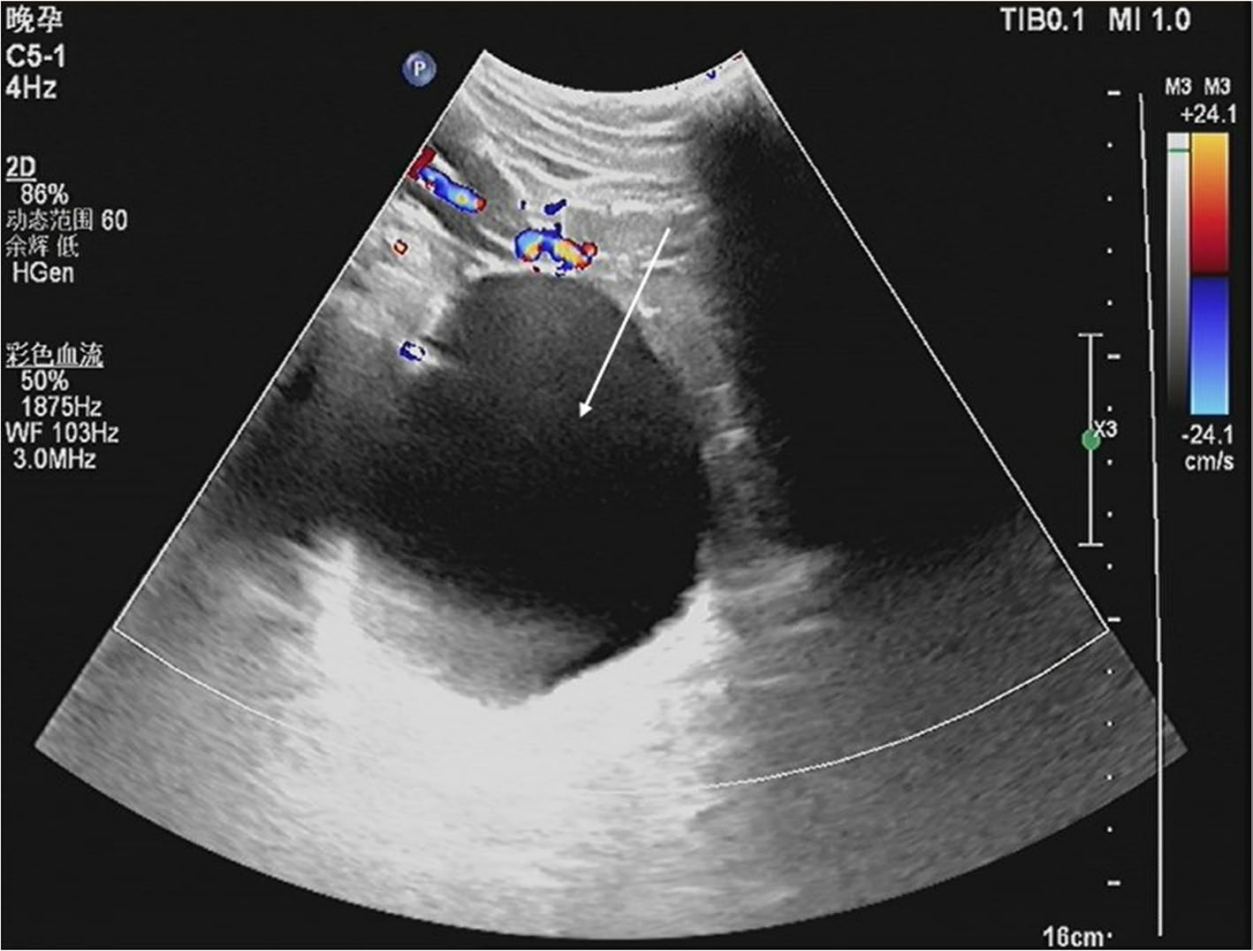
Sonography at 34 weeks of gestation. A large pelvic hydatid cyst is shown (9.5*8.6 cm) (arrow)
